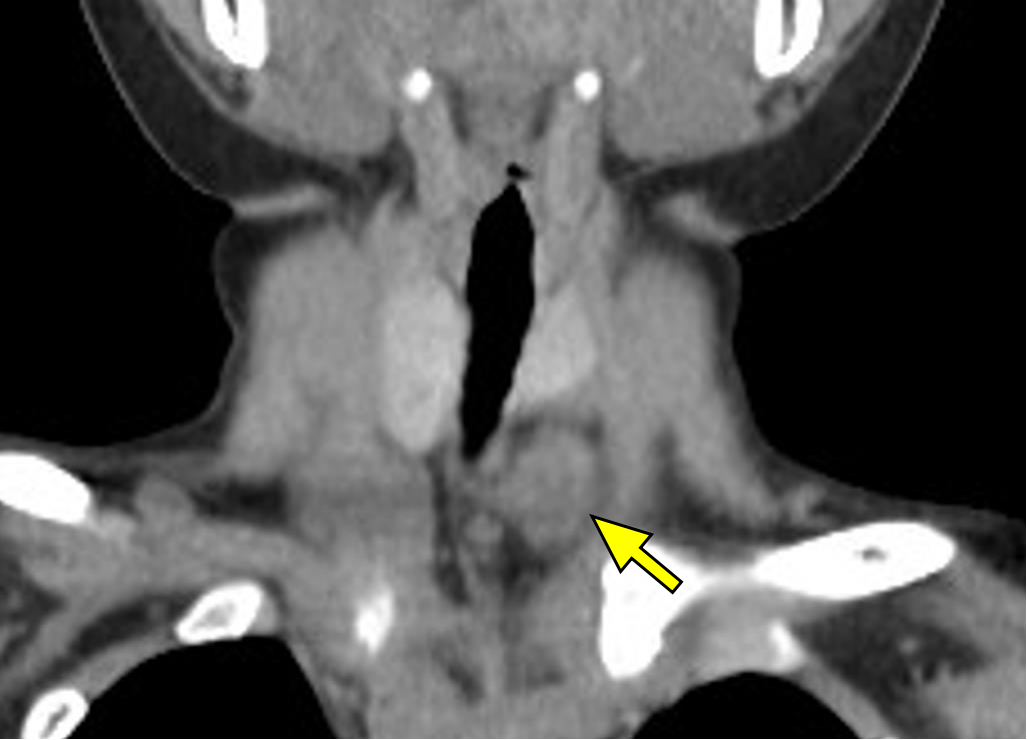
Preoperative contrast-enhanced CT scan showing a 4-cm mass (arrow) near the inferior pole of the left thyroid lobe.
